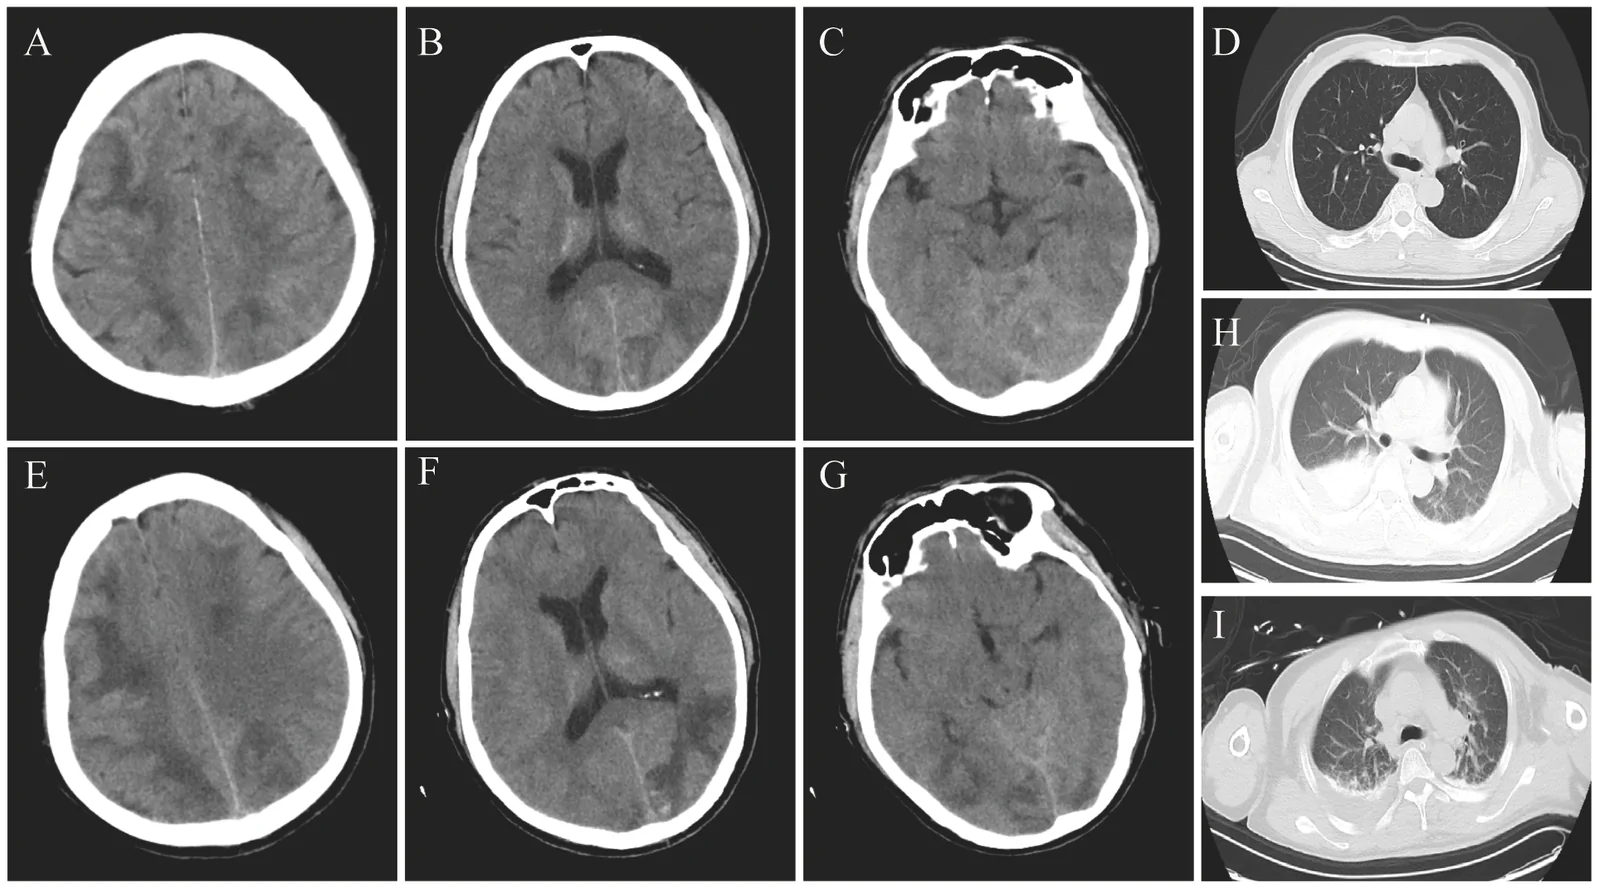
Postoperative follow-up imaging. (A–C) Cranial CT on postoperative day 1 showing decreased intracranial hyperdense Lipiodol deposits. (E–G) Cranial CT on postoperative day 3 showing further clearance of deposits while ischemic changes persisted. (D) Preprocedural comparison image before the fourth interventional treatment. (H, I) Chest CT on postoperative days 1 and 3 showing right lower-lobe atelectasis, pleural effusion, and iodized-oil deposition in the treated hepatic region.
